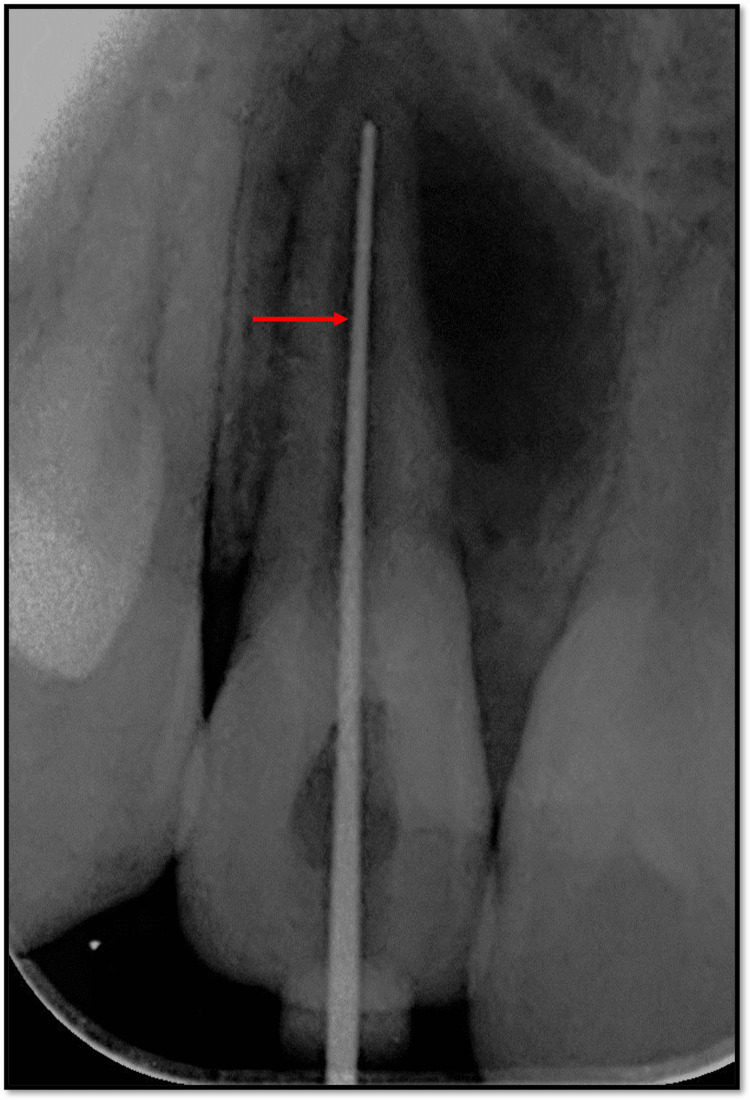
Radiographic confirmation of working length with 12
